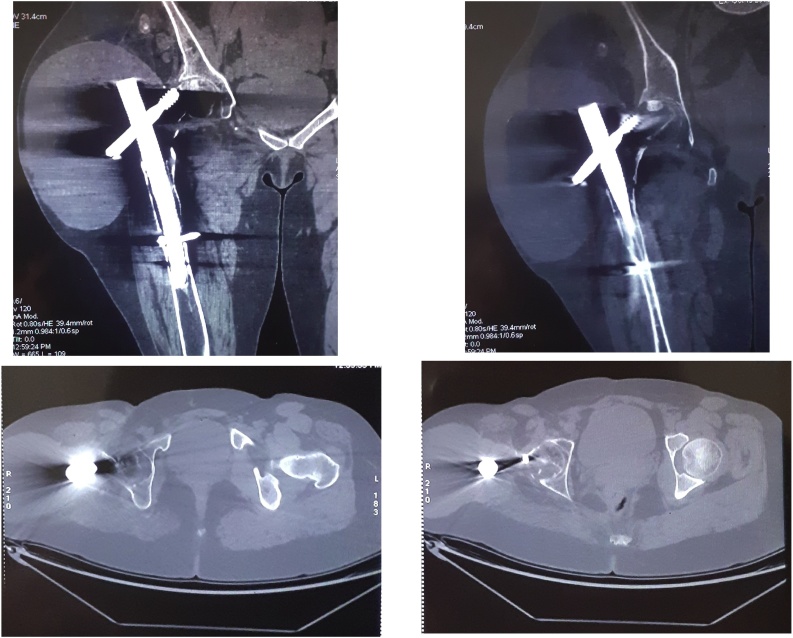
CT images showing a bone loss in the trochanteric region, and cystic lesion of the peri-trochanteric soft tissue..
